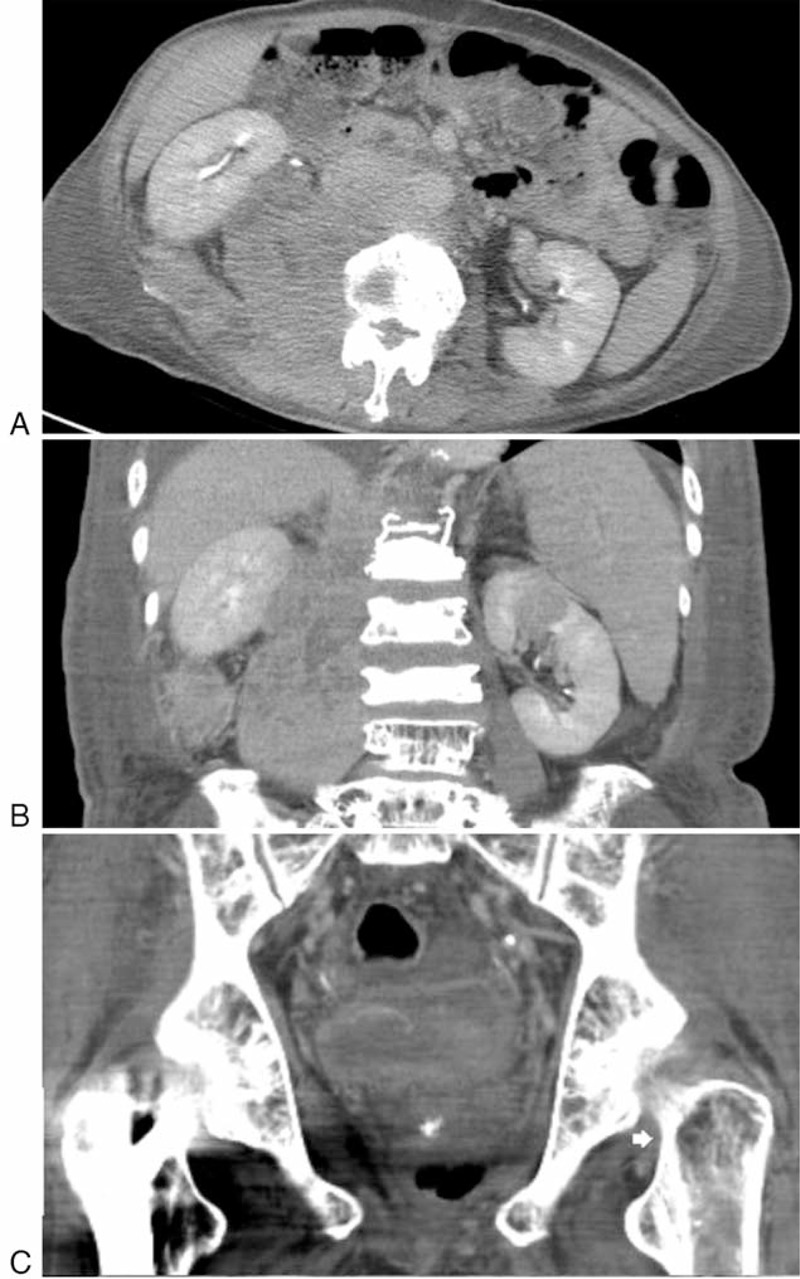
Abdominal CT following the Ga-67 scan. (A) Transverse and (B) coronal views showed a large right iliopsoas mass extending to the right peri-renal, posterior pararenal and prevertebral space, and (C) an obvious osteolytic lesion at left proximal femur (arrowhead). Biopsy from the right paraspinal region proved plasma cell myeloma, indicating extramedullary disease.
